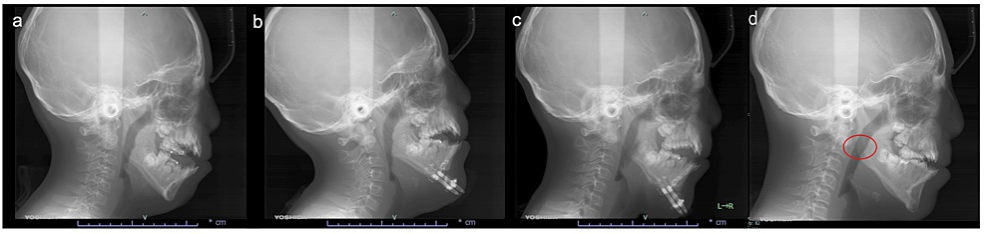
Pre- and postoperative X-rays. a) Preoperative X-ray. b) Pre-distraction X-ray. c) Post-distraction X-ray. d) Two years after surgery, showing obvious airway expansion (red circle).
